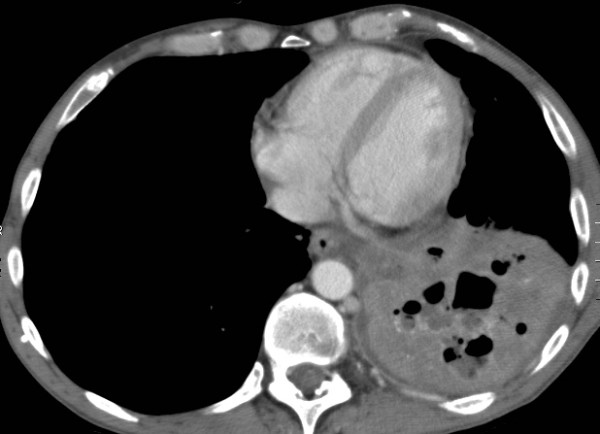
Axial CT image shows a left lower lobe consolidation. Irregular fluid- and gas-containing collections are seen in keeping with cavitation.
